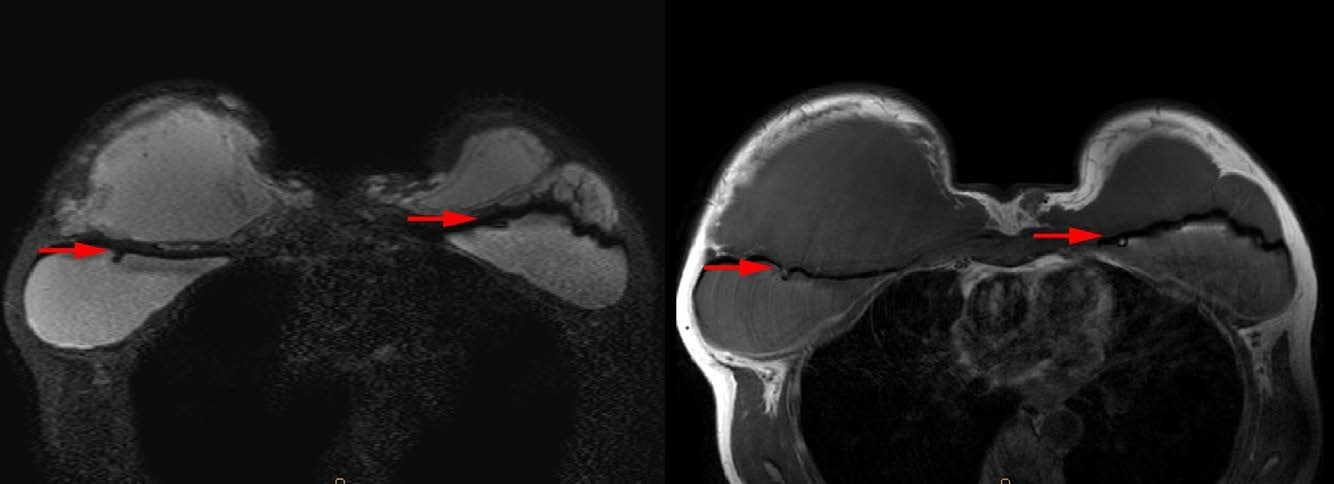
Magnetic resonance imaging. “Tear drop sign” of both implants could be seen on T1 and T2 imaging (red arrows), suggesting suspicious rupture of the implants.
